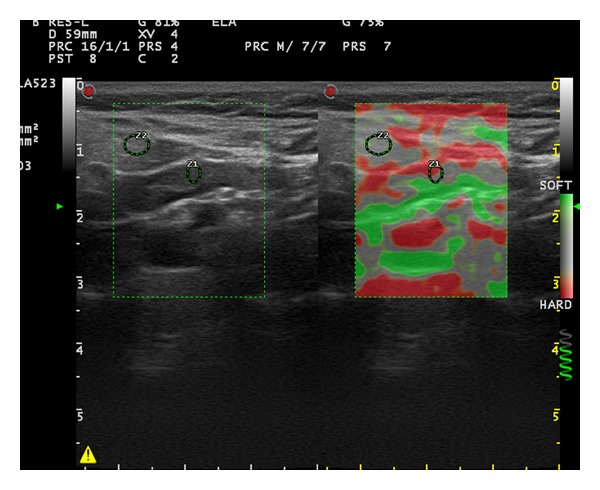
Elastosonography of the cervical lymph node on the right side of a 50-year-old female patient (long axis: 24.9 mm, short axis: 5.2 mm, cortical thickness: 3.5 mm, hilar thickness: 1.8 mm, strain ratio: 2.03, and elasticity score: 2).
